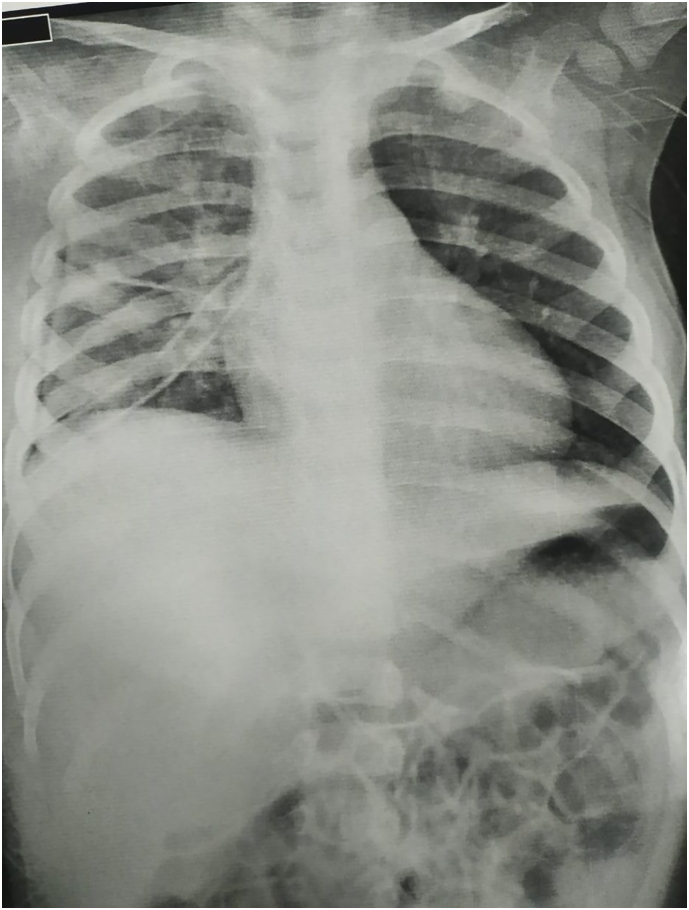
Follow up chest x-ray showing chest tube in place.
